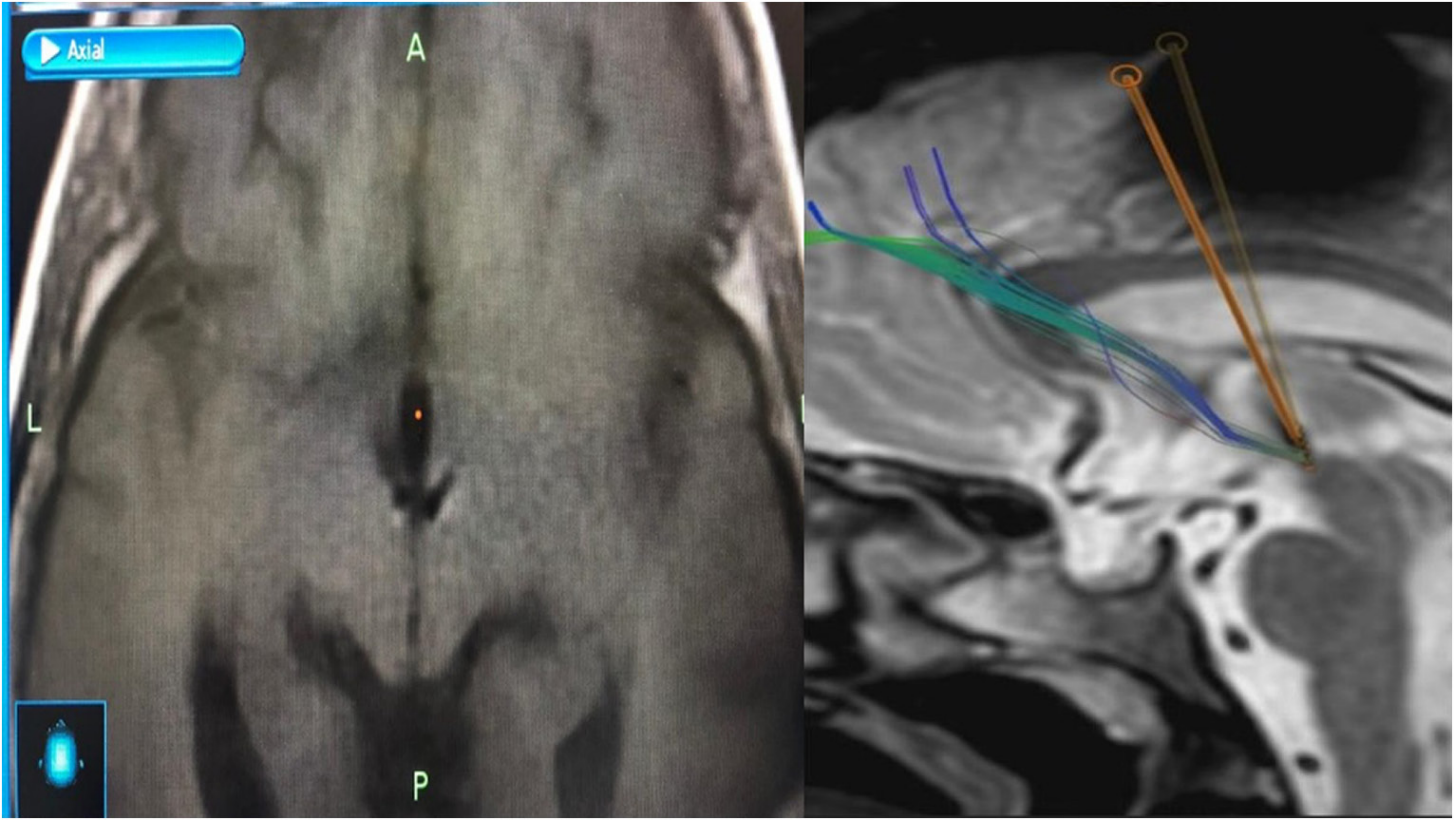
Tw1 MRI/CT‐S intraOp fusion showing deep brain electrodes implanted in the pHypNs.
